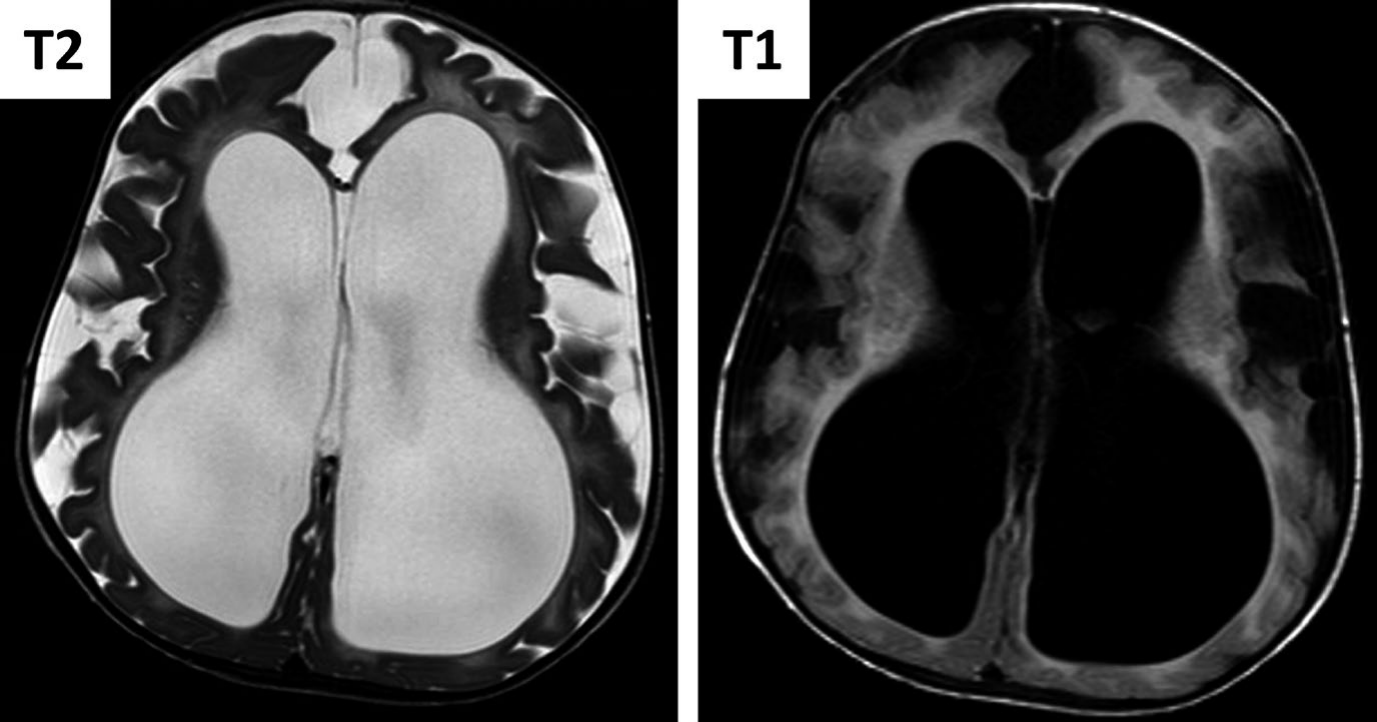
Brain MRI of patient V5 at 21 months. T1 (Right) and T2 (Left) weighted MRI showing white and gray matter atrophy with secondary enlarged lateral ventricles
